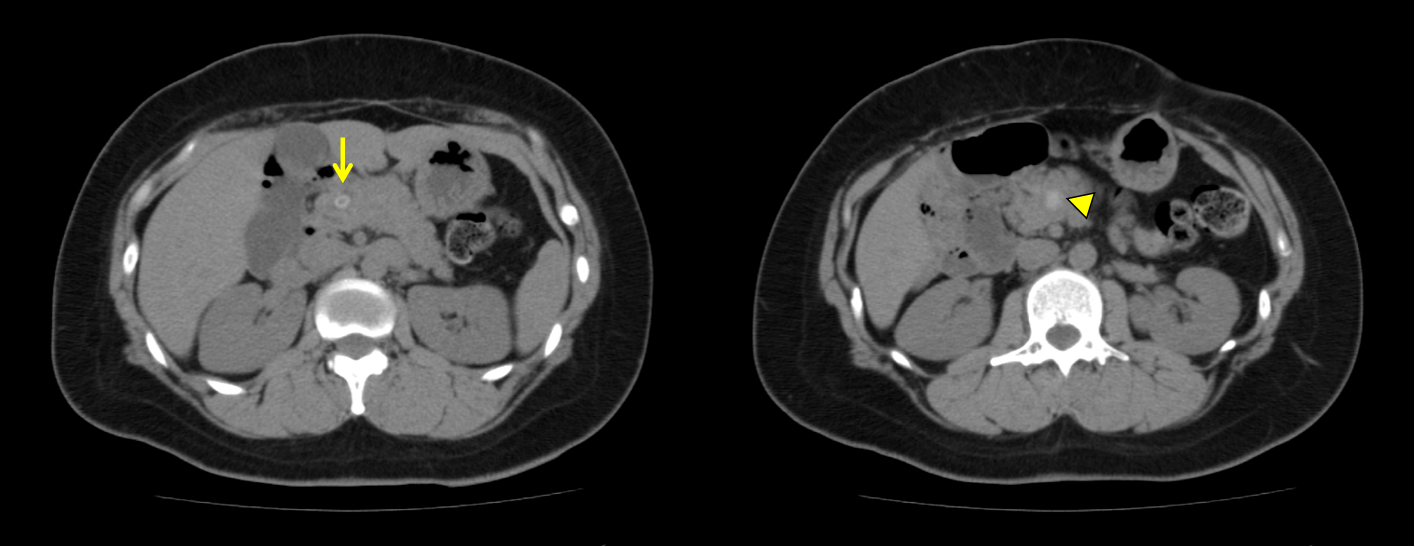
Findings of computed tomography. The choledocholithiasis (arrow) and pancreatic calculi (triangle) was detected
